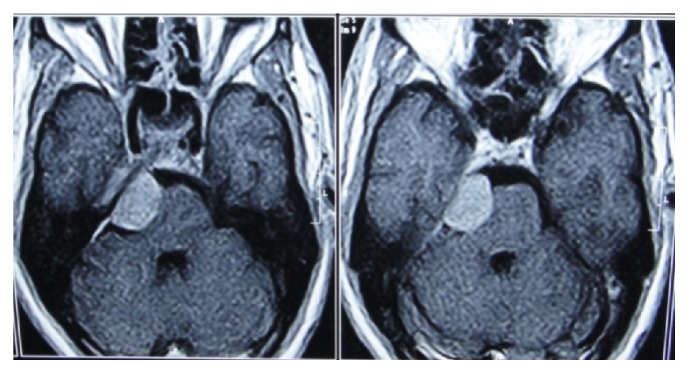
MRI T1W axial image with contrast shows well-defined intensity enhancing extra-axial mass lesion involving right cerebral pontine angle region. MRI impression: MRI study in contrast revealed possibility of CP angle Schwannoma or Meningioma.
